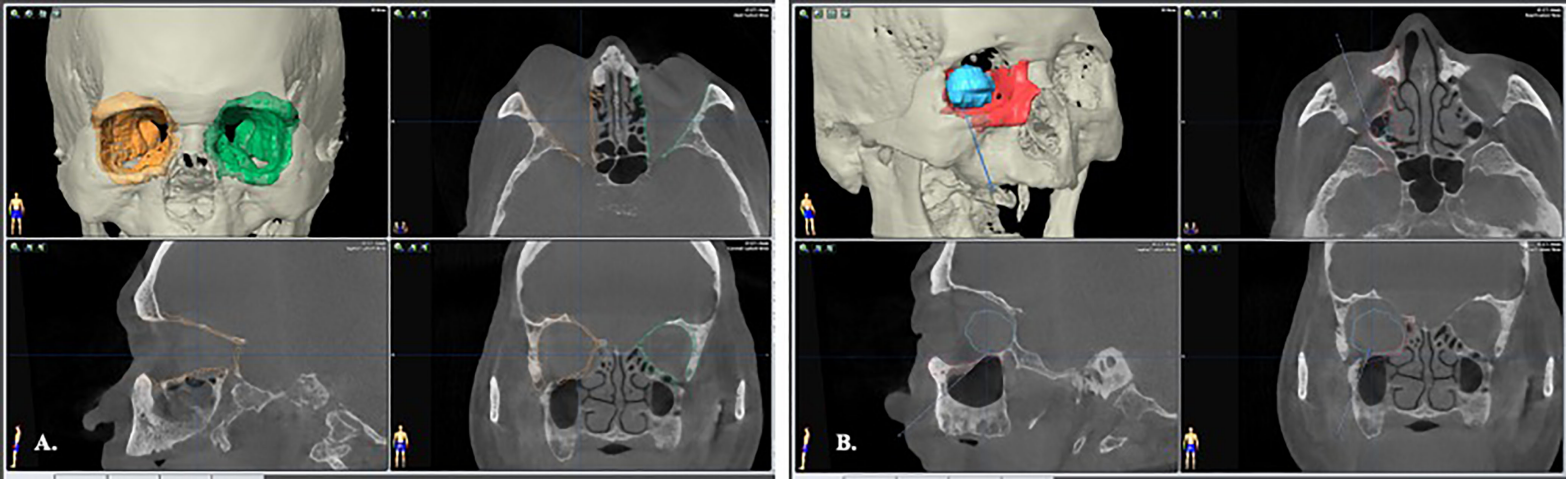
Image segmentation by bone-specific Hounsfield units. (A) segmentation of the normal, non-destructive orbital wall (green); the mirrored image from the contralateral normal orbital wall to rebuild the destructed right side medio-inferior orbital walls (orange). (B) The orbital tumor was segmented with fusion MRI and CT images to optimize the tumor boundaries (blue). A trajectory through the maxillary sinus that indicated the path of entry to orbital tumor was designed for intraoperative guidance.
